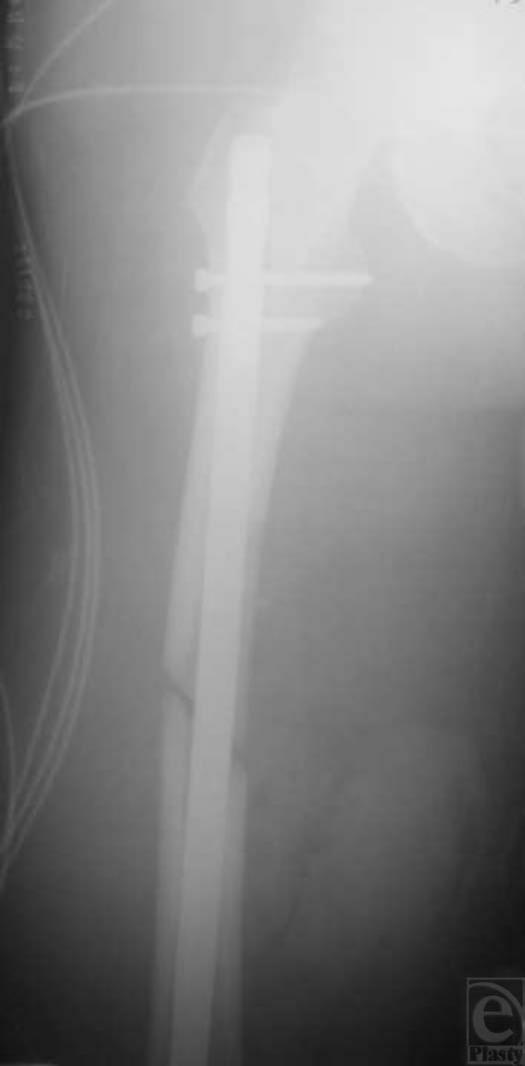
Postoperative anteroposterior radiograph shows reduction and intramedullary fixation of the right femur.
